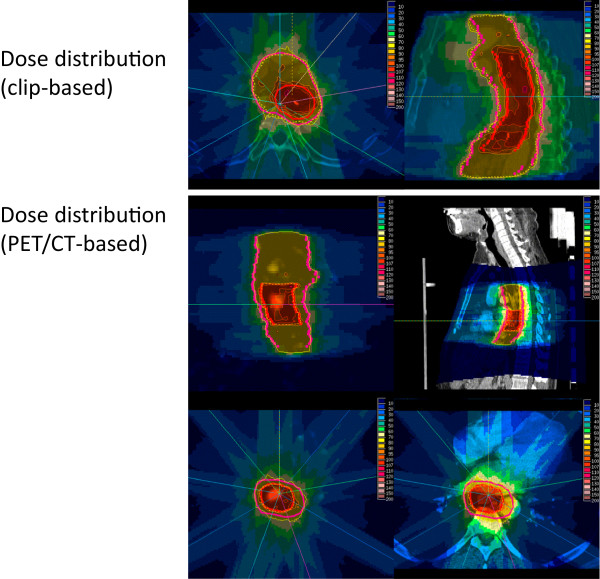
Example pictures for dose distributions. Sample pictures showing dose distributions as obtained from clip-based (upper panel) or PET/CT-based treatment planning. Red lines define boost volumes and pink lines planning target volumes.
